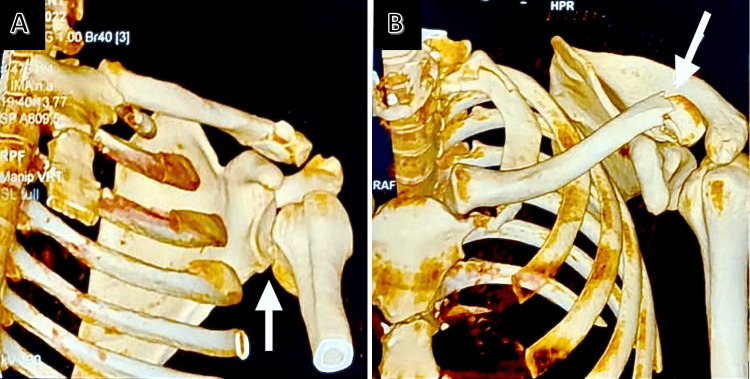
CT scan of the left shoulder showing (A) the joint dislocated toward the back, and (B) a fracture in the outer part of the clavicle.
